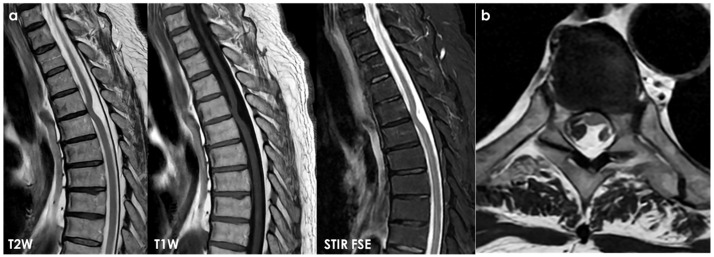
Spinal dorsal MRI in sagittal (a) and axial T2W (b) sections, showing a classical “scalpel sign”. The diagnosis of SAW was surgically confirmed.
